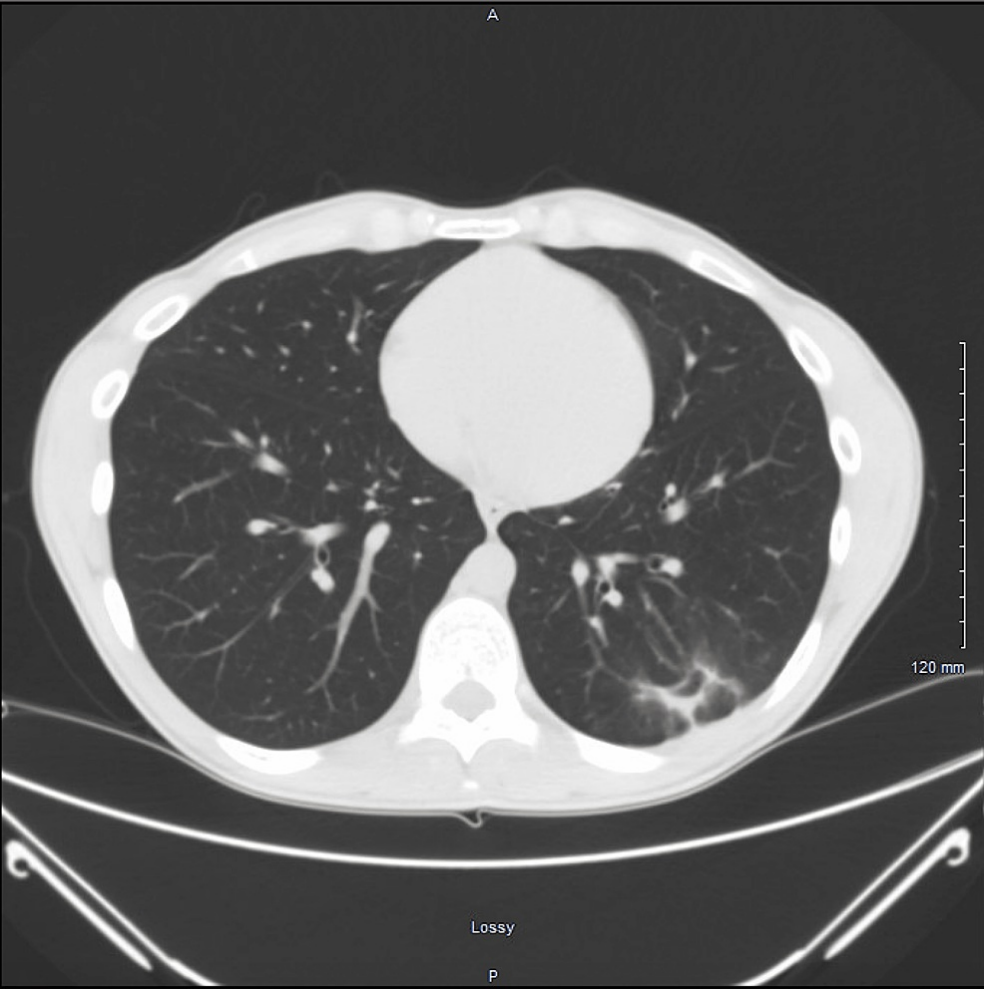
Transverse computed tomography image showing resolution of the lung mass with residual cavitation in its place
